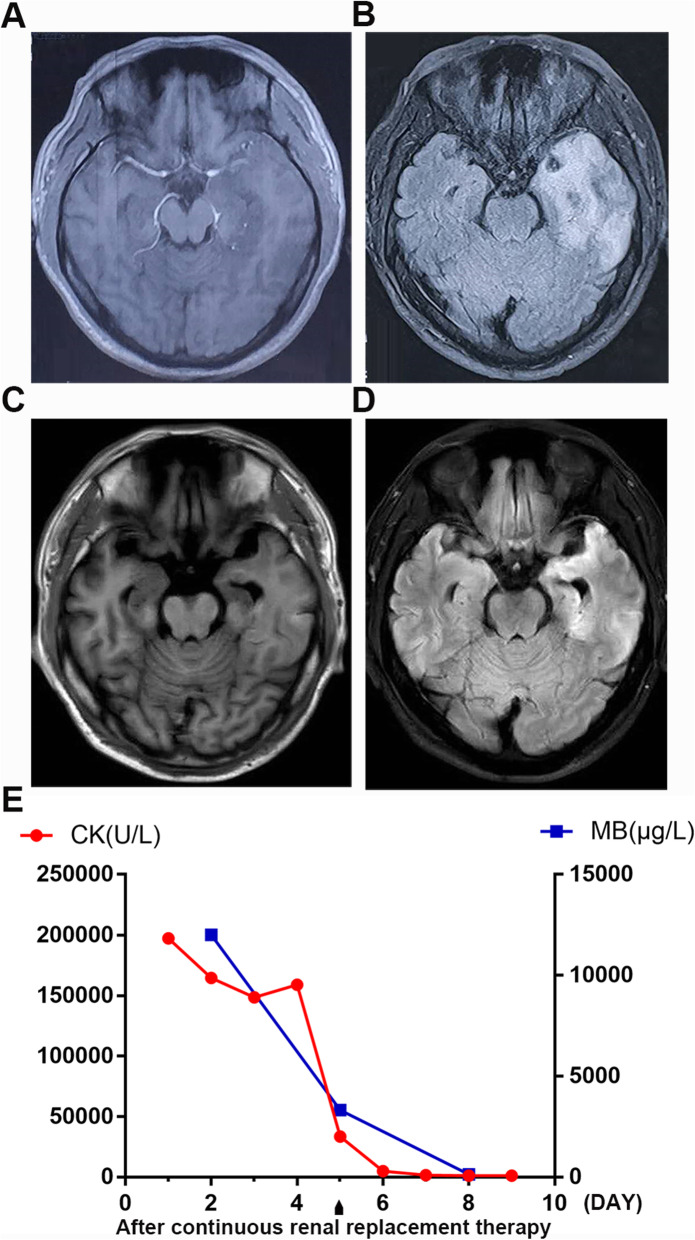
the changes of MRI images and content of MB and CK before and after treatment. a and b The left temporal lobe, hippocampus and insula were swollen as seen on the MRI image and high T2 signal was seen in these areas upon hospitalization. c and d MRI images taken at follow-up revealed significant improvement of the intracranial lesions. e The trend of Mb and CK of the patient before and after treatment of hemodialysis. Mb, myoglobin. CK, creatine phosphokinase
